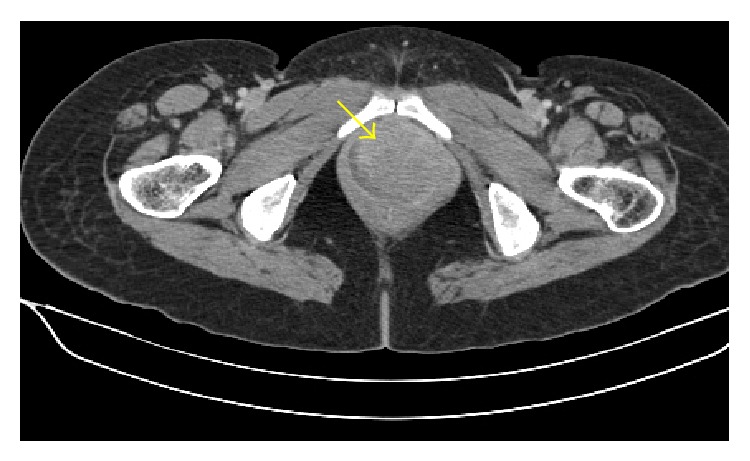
Computed tomography (CT) image of the pelvis shows well-defined homogenously enhancing lesion which is arising from left sided anterolateral wall of the vagina and projecting into vaginal lumen.
